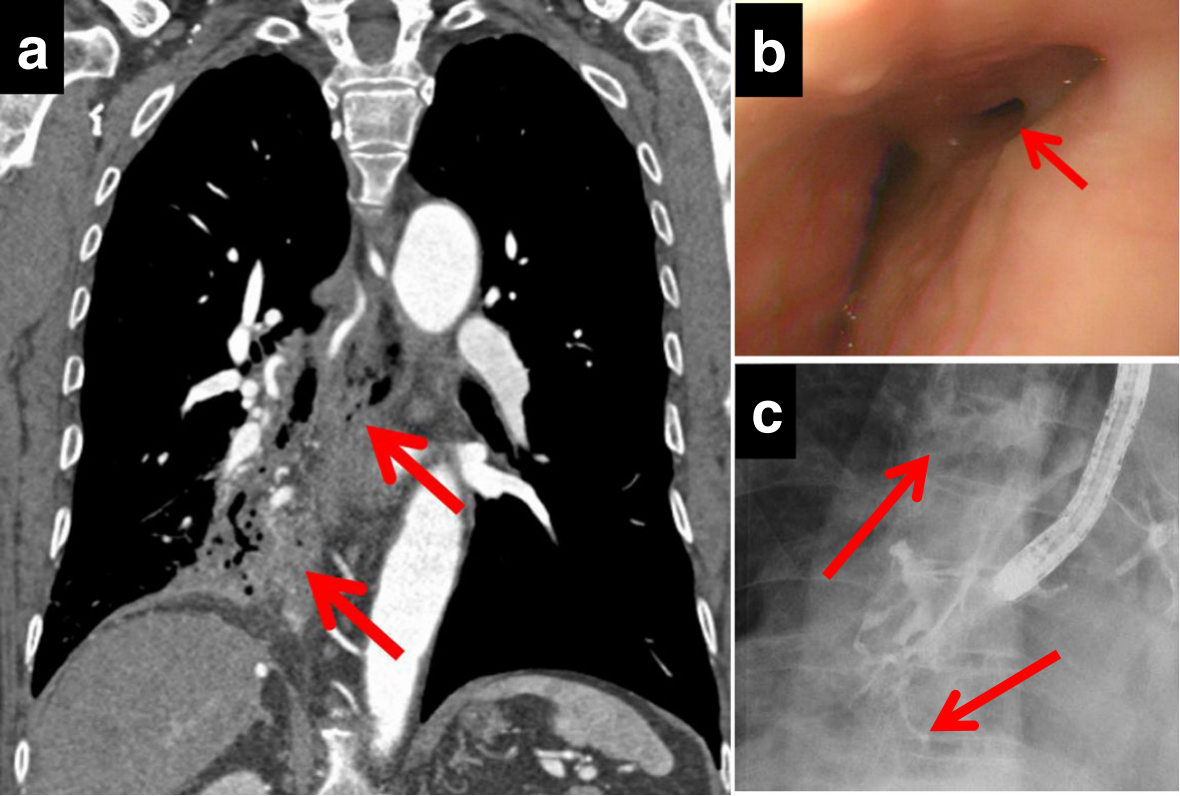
a Chest contrast-computed tomography. A connection (*arrows*) between the middle of the esophagus and the right lower lung lobe was suspected. b Esophagoscopy findings. A diverticulum-like depression was seen (*arrow*) at the 2 o’clock position on the wall of the middle esophagus. c Esophagography findings. The flow of the contrast medium was detected (*arrow*) from the esophagus depression to the right lower lung and finally to the right main bronchus
